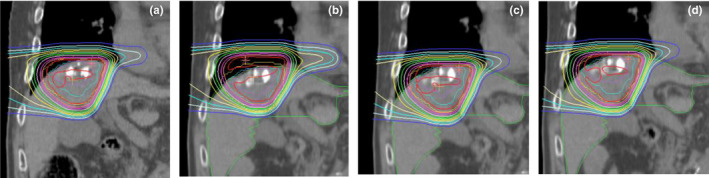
Dose distributions on coronal image for lesion number 1 where there was a displacement of right diaphragm of 10‐mm inferior direction: (a) reference dose distribution on the initial planning computed tomography (1st‐CT), (b) recalculated dose distribution on the ongoing verification CT (2nd‐CT) using vertebral body matching, (c) recalculated dose distribution on 2nd‐CT using diaphragm matching, (d) recalculated dose distribution on 2nd‐CT using marker matching. The dose distribution is displayed on a graduated scale from 10% to 100%.
